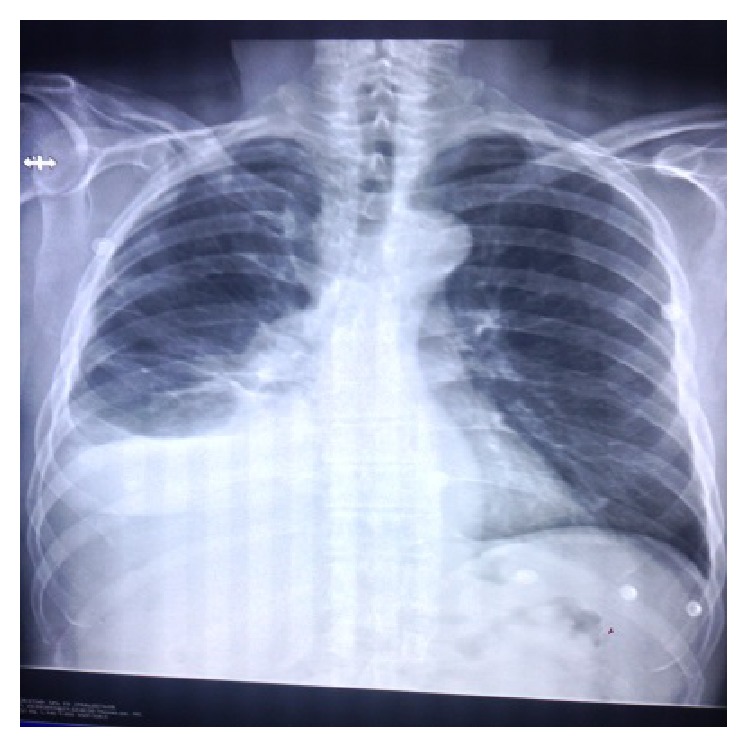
Chest X-ray of 73-year-old male revealing large right unilateral pleural effusion.
